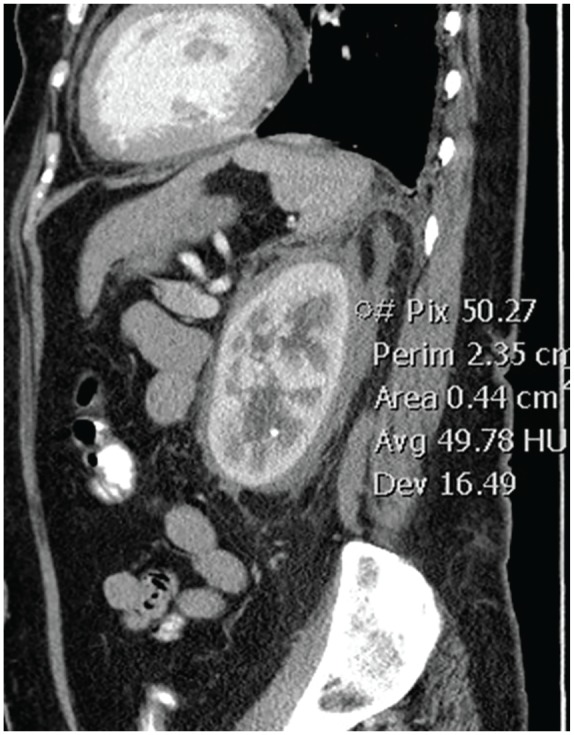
Sagittal computed tomography image through the left kidney with the circled region of interest demonstrating a density measurement of 49.78 Hounsfield units, confirming soft tissue infiltration of the perinephric space.
